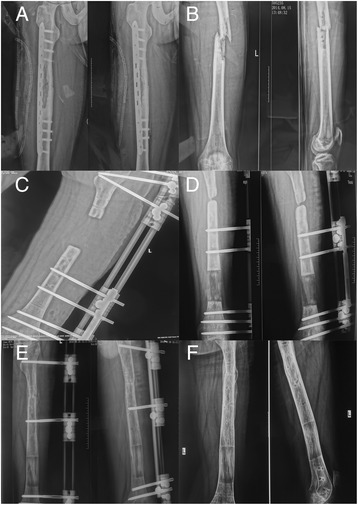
Twenty six-year-old male, suffered a 14-month-long infection after the operation using bone plates of fracture of left femoral shaft. a The X-ray presentation after the open debridement combined with irrigation outside the hospital. b After removing the internal fixator due to the runaway infection, the postoperative presentation of the X-ray demonstrated the nonunion of fracture and evident displacement, and some sequestra with bone defects could be seen locally. c The 1-week-later X-ray presentation after bone transport: single-arm external fixator served well, and there existed a 6-cm-long bone defect after thorough removal of sequestra and infectious tissues. d The 2-month-later X-ray presentation after bone transport: bone growth could be seen in the region of distraction osteogenesis, but both sides of docking site were significantly hardened. e The 14-month-later X-ray presentation after bone transport: the docking site healed well after debridement, autogenous bone graft, and compression. The bone grew well in the region of distraction osteogenesis and external fixation pins were partly removed. f: The 20-month-later X-ray presentation after bone transport: no infection recurred
